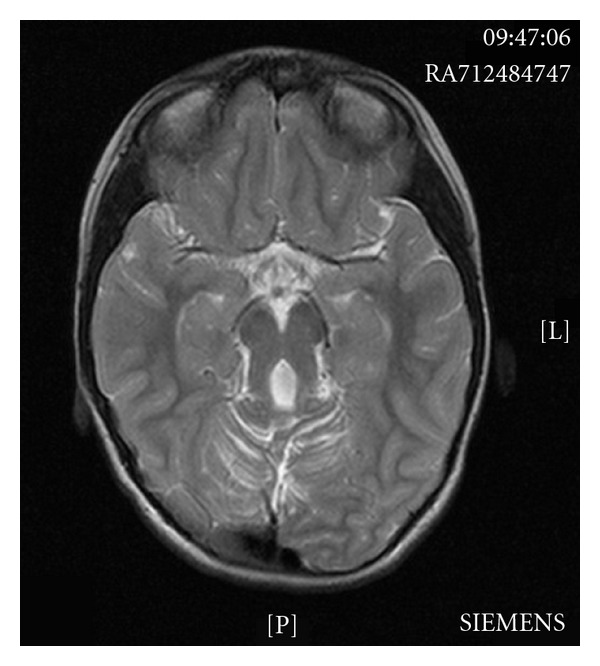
Cranial T2 magnetic resonance image for AZ—this shows a midline defect in the cerebellar vermis, thickened horizontally orientated cerebellar peduncles, a small midbrain, and dysplasia of the cerebellar cortex. This demonstrates the characteristic “molar tooth sign” seen in Joubert Syndrome and JSRD.
